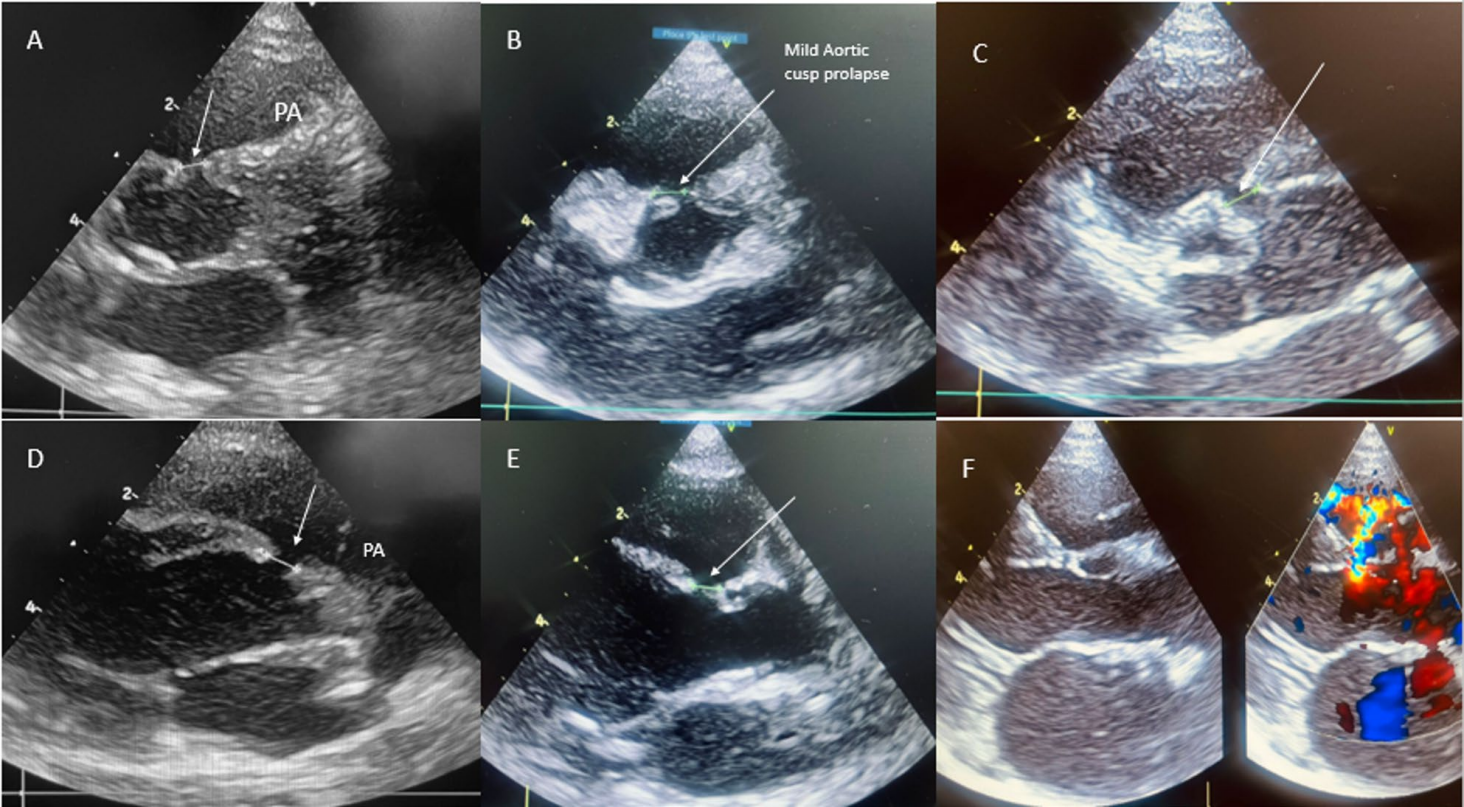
A-E showing modified parasternal short axis for complete profiling outlet muscular defect with varying degree of aortic valve prolapse and prominent muscular rim with arrow depicting defect and cusp prolapse, F showing outlet muscular defect in parasternal long axis view with colour Doppler interrogation
